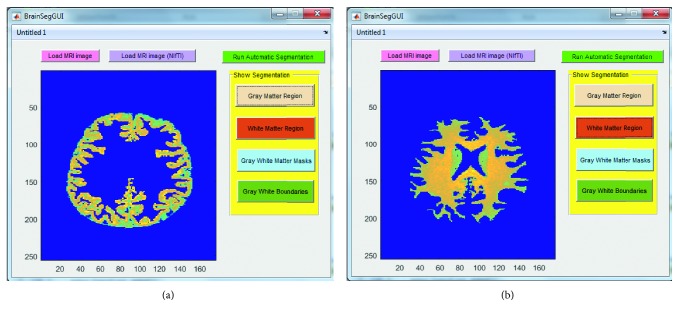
Screenshots of the graphical user interface (GUI) designed and developed in this work for automatic brain MRI image processing. Steps shown here are to show extracted gray (a) and white (b) matter regions upon the click of the “Gray Matter Region” (a) and “White Matter Region” (b) buttons, respectively.
